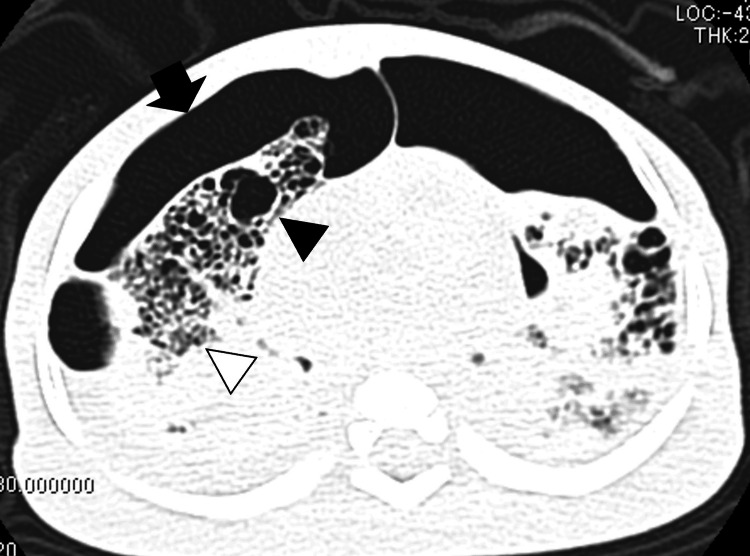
Postmortem CT findings The air leak is shown in the black arrow. Cystic change is shown in the black head. Fibrotic change is shown in the white head. CT, computed tomography
